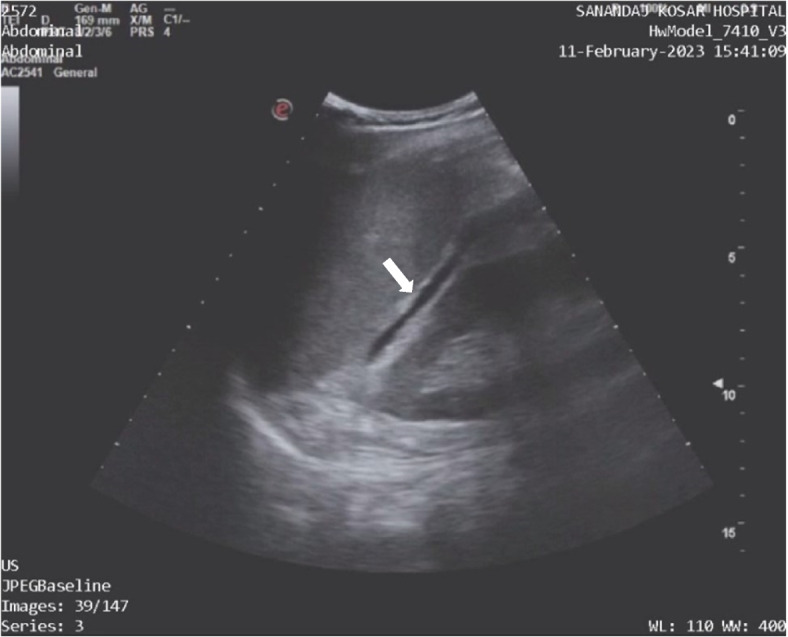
Ultrasound showing perinephric fluid
